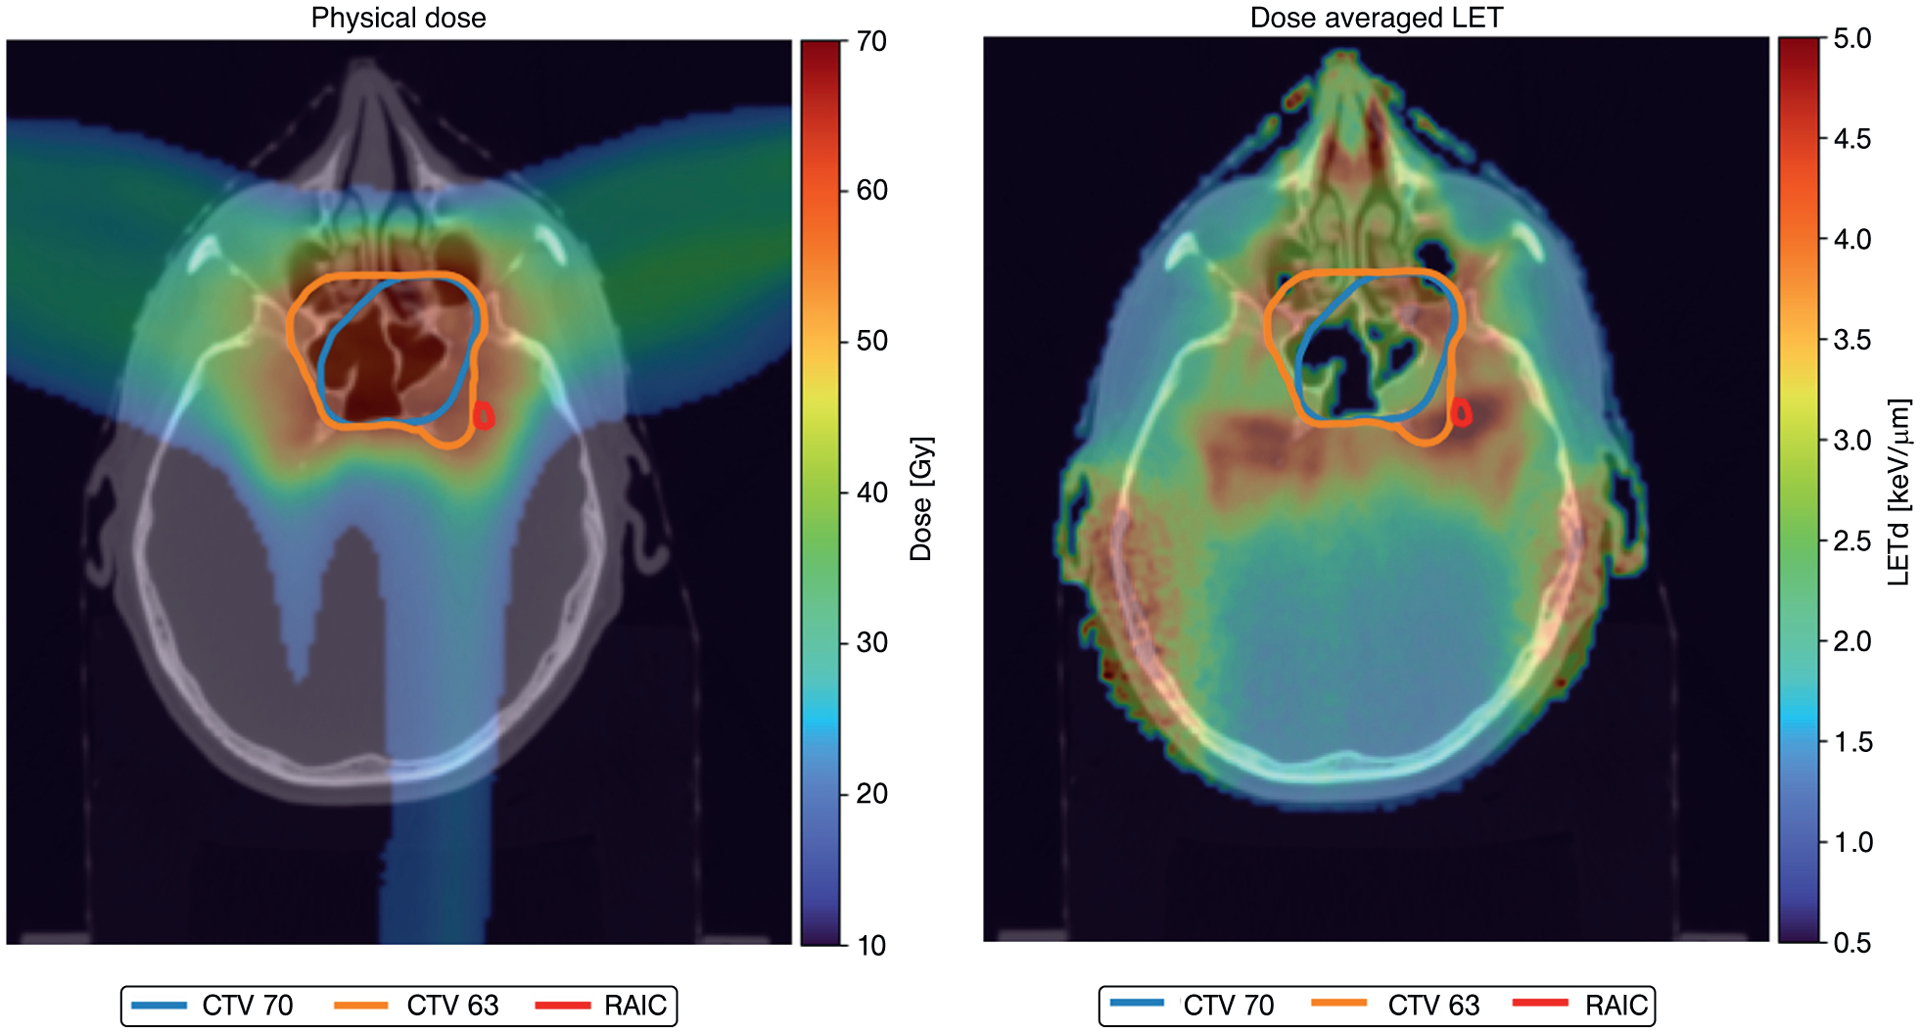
Example of dose distribution (physical dose) to the left and the dose-averaged linear energy transfer (LET_d_) distribution to the right. The high LET_d_ in low-dose regions laterally are due to secondary radiation. Radiation-associated brain image change (RAIC) (red contour): the contoured contrast enhanced lesion from the T1 weighted magnetic resonance imaging (MRI).
